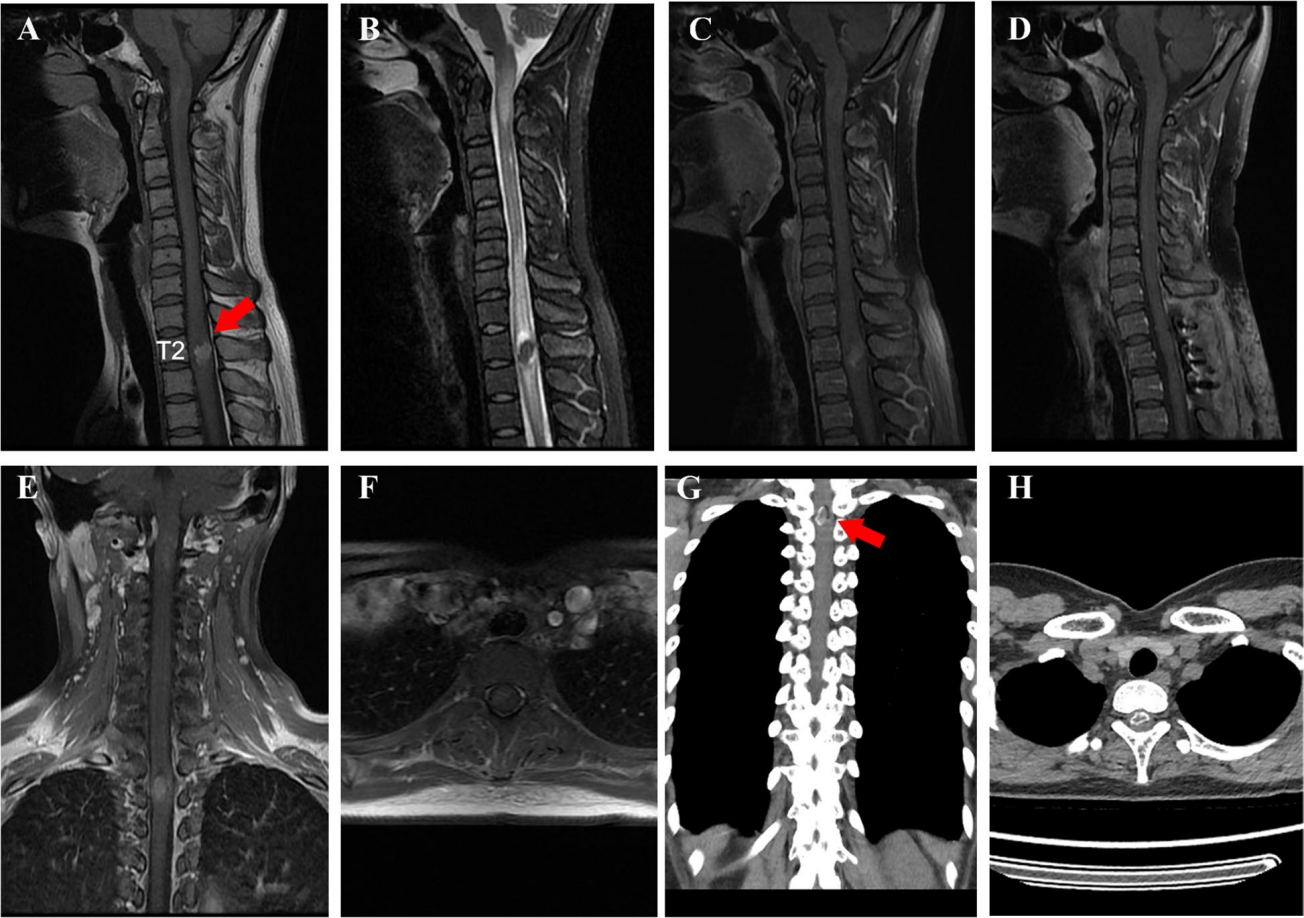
MRI and CT findings. Preoperative MRI revealed an irregular hyperintense mass in sagittal T1 (A) and hypointensity in T2 (B). The lesion was solid with hemorrhage in sagittal (C) and coronal (E) imaging after administration of gadolinium and was intramedullary on axial enhanced MRI (F). Postoperative contrast-enhanced MRI showed the tumor was totally resected (D). Preoperative CT depicted high calcification the hyperdense mass (G, H). Red arrow: location of the tumor
